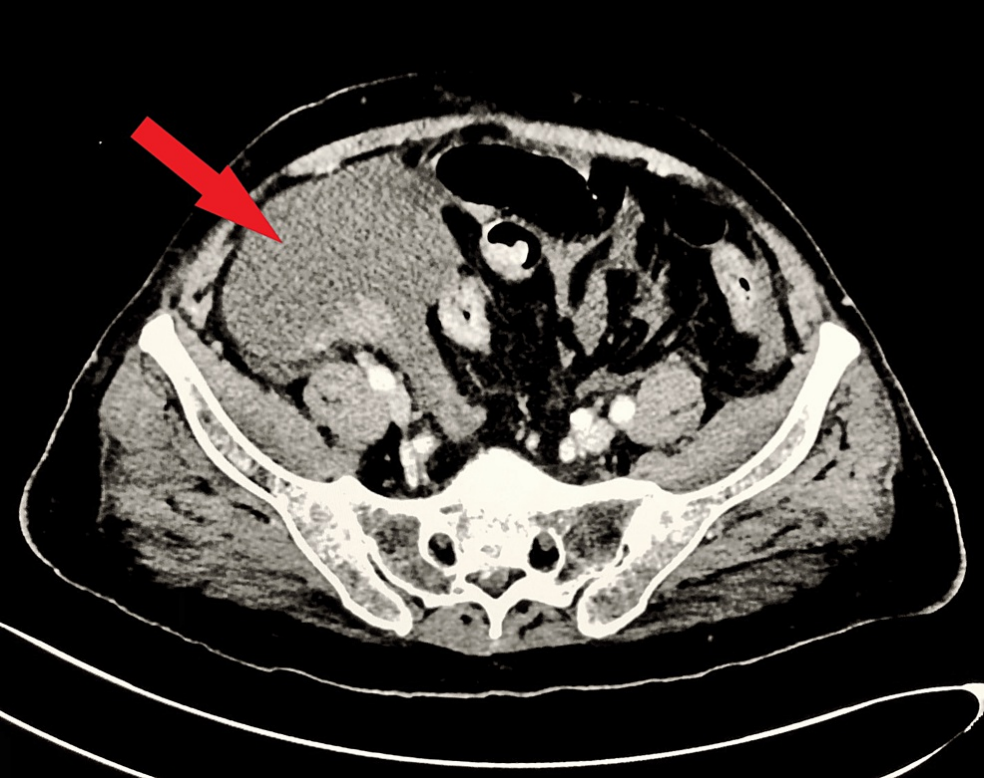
Axial computed tomography (CT) scan of the pelvic region revealing hemoperitoneum (arrow)
